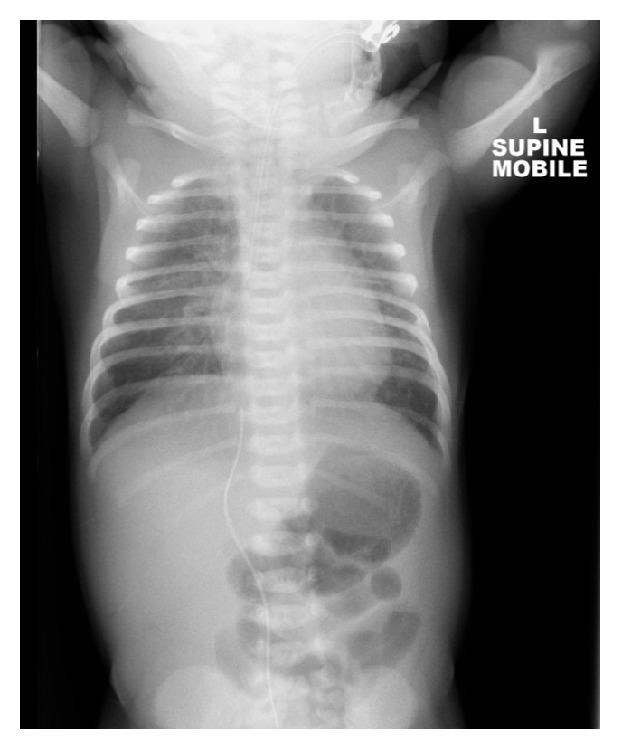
Chest X-ray and abdominal X-ray at two hours of age. The endotracheal tube tip is in the right main bronchus and was withdrawn 15–20 mm. The cardiothalamic silhouette is normal and lung and pleural spaces are clear. No pneumothorax is seen. The umbilical venous catheter lies to the right of the midline at T10.
